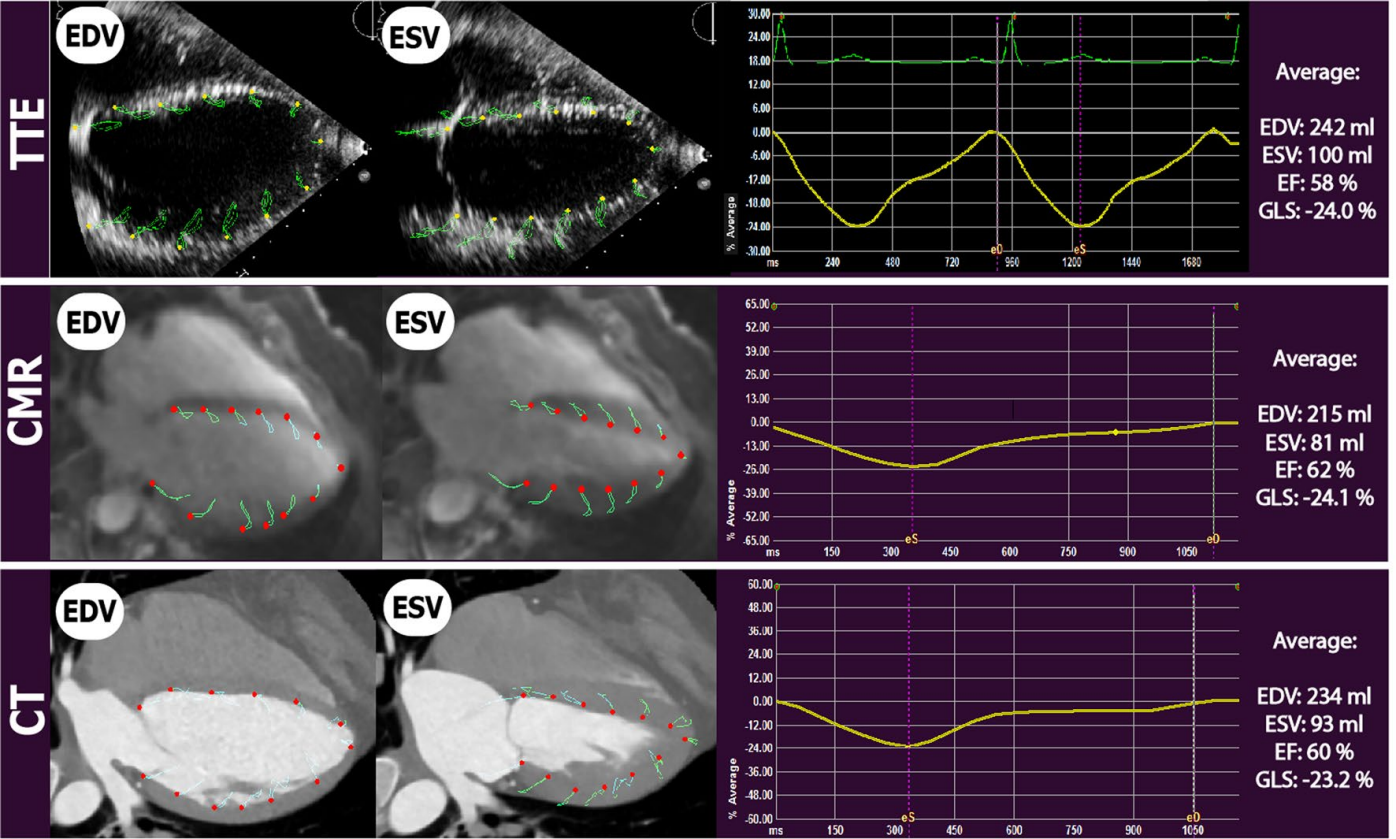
Left ventricular parameters by three different modalities in the same patient. *TTE* Transthoracic Echocardiography, *CT* Computed Tomography, *CMR* Cardiovascular Magnetic Resonance, *EDV* end-diastolic volume, *ESV* end-systolic volume, *EF* Ejection Fraction, *GLS* Global Longitudinal Strain. Yellow lines depict GLS during the cardiac cycle. *eS* end-systolic phase, *eD* End-diastolic phase
